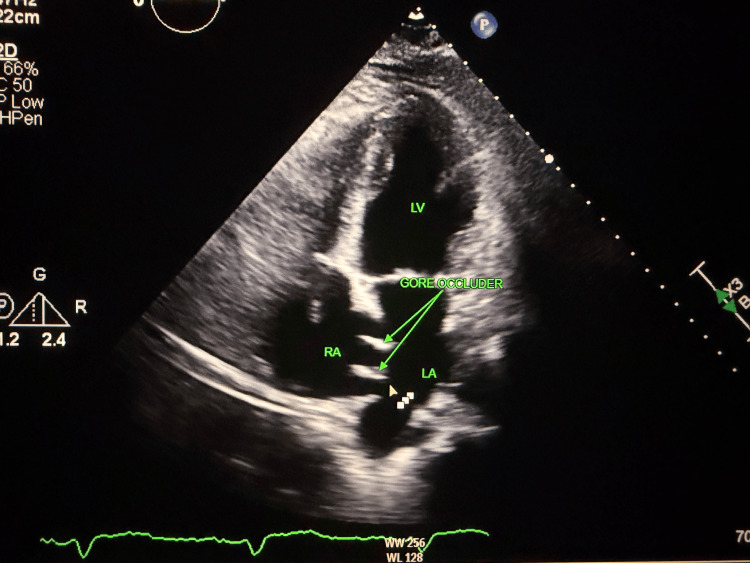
TTE After Deployment of a 30 mm Gore Occluder for PFO Closure TTE: transthoracic echocardiogram, PFO: patent foramen ovale.
